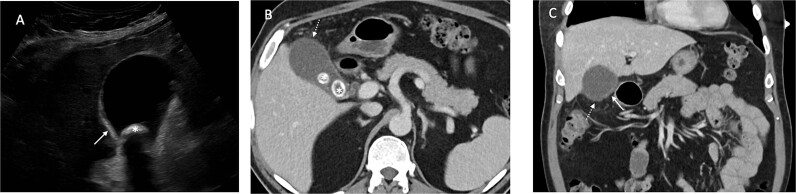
Ultrasound demonstrates gallbladder wall thickening (solid white arrow in A) and gallstone (* in A). Contrast enhanced CT of the abdomen in portal venous phase axial (B) and coronal (C) planes demonstrate gallbladder wall thickening (solid white arrow in C) and area of nonenhancing wall (dashed white arrow in B and C) and gallstones (* in B).
